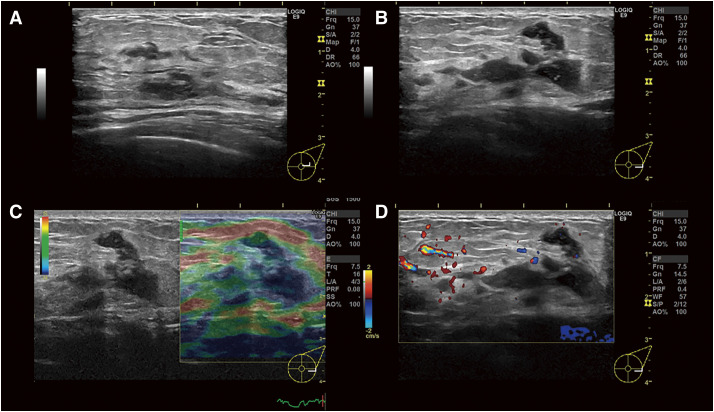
Ultrasonographic imaging findings in a case of cystic neutrophilic granulomatous mastitis caused by *Mycobacteroides abscessus*. (A and B) Ultrasonographic images showing an irregular hypoechoic area extending over the map to replace the mammary tissue in the left breast at the left 3 o’clock position. The hypoechoic area extends partially to just below the skin. (C) The Tsukuba elasticity score was 5, suggesting malignancy. (D) Color Doppler image showing increased blood flow signals around dilated ducts.
